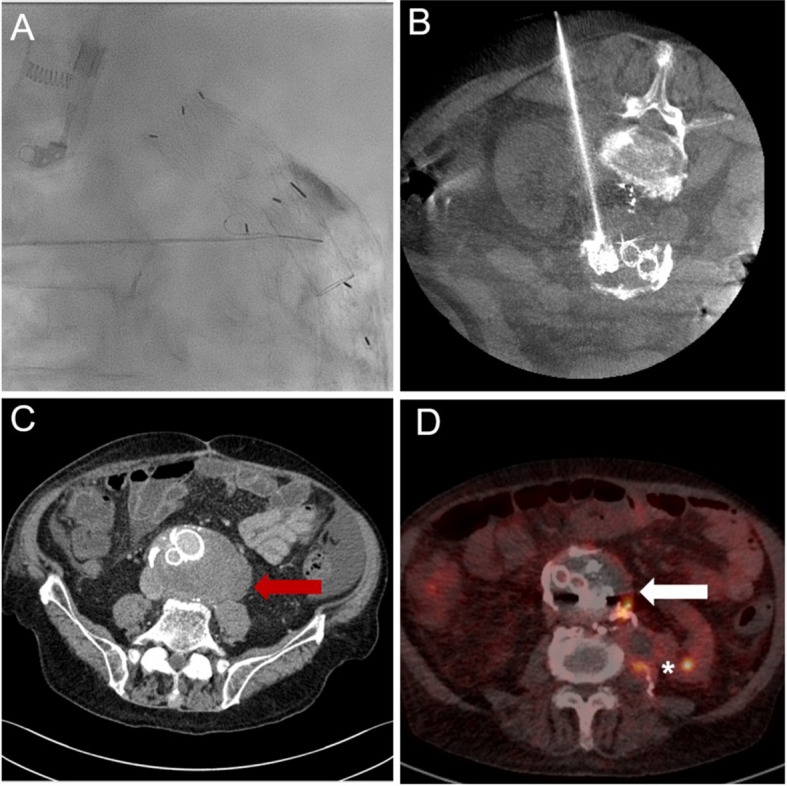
Representative case of a 78-year-old male patient undergoing a second attempt of type II endoleak embolization via direct sac puncture from the third right lumbar artery using EVOH. A, B Intra-procedural images of the embolization. C Follow-up CT 33 days after the procedure showing a circumferential periaortic soft-tissue mantle in the presence of clinical symptoms and elevated inflammatory markers. D FDG-PET/CT performed 11 days later demonstrating increased tracer uptake along the aortic wall (white arrow) and ring-shaped uptake within the left psoas muscle (white asterisk), consistent with abscess formation subsequently confirmed by CT
